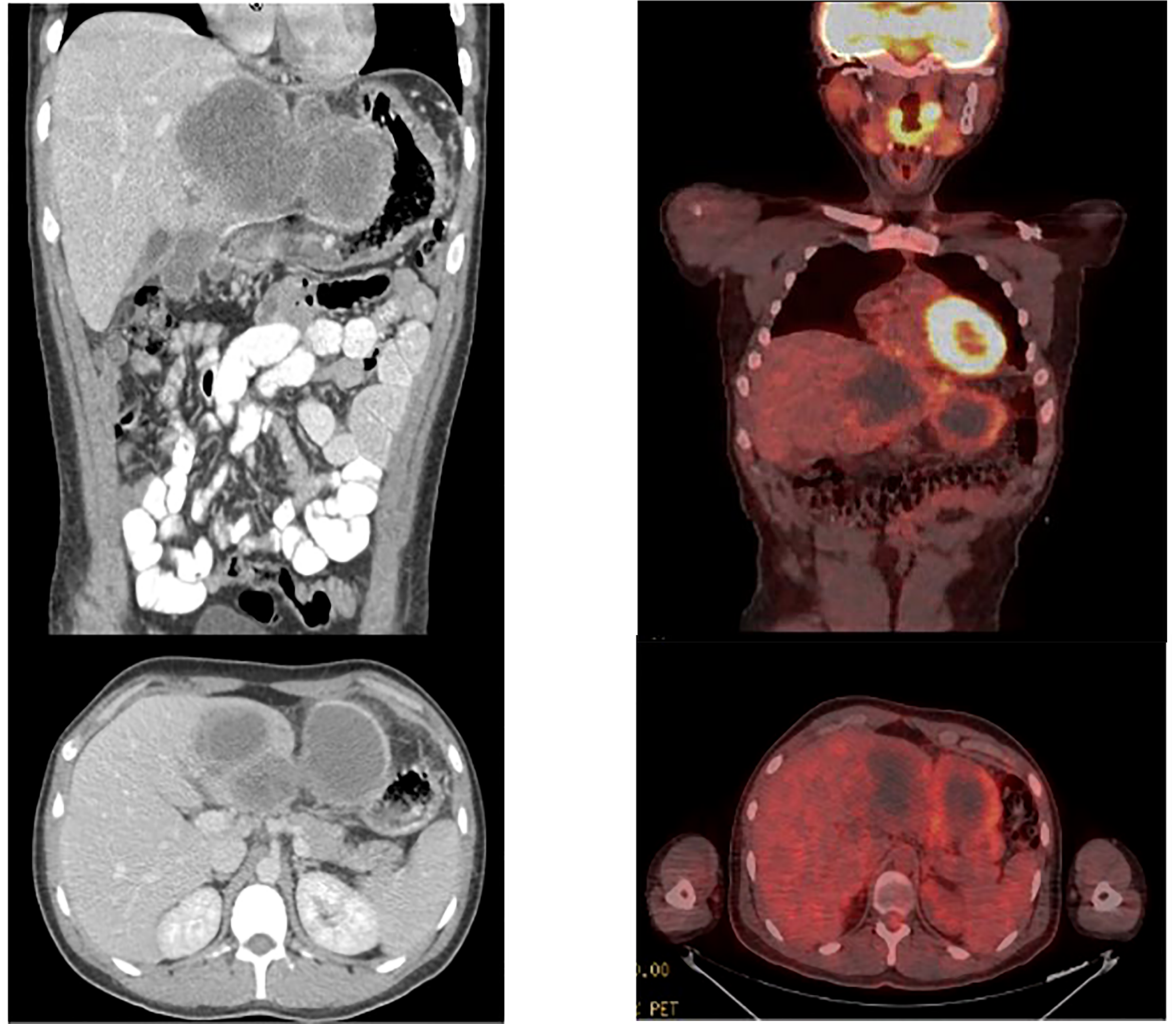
CT scan after completion of neodjuvant chemotherapy. Significant improvement was visualized with a decrease in lesion size to 15 x 7.7 x 8.1 cm. PET scan after completion of neodjuvant chemotherapy demonstrating reduction in size of mass to 14.5 x 6.8 x 8.9 cm as well as decreased FDG avidty of SUV 5.4.
